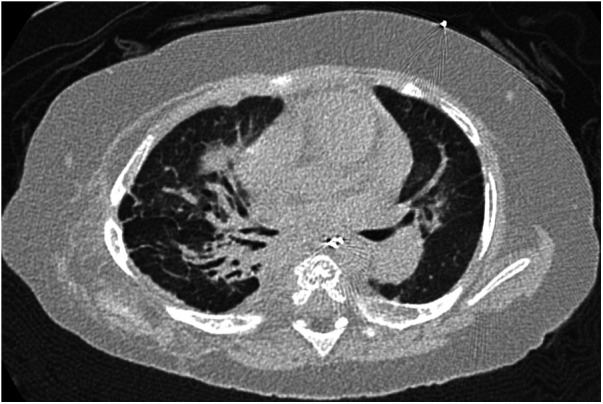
Case 2: axial CT images of lung window settings at 1 year of age. Axial CT images show parahilar consolidation and subpleural linear opacities of atelectasis or fibrosis with bronchodilation, predominantly in the right lung field.
